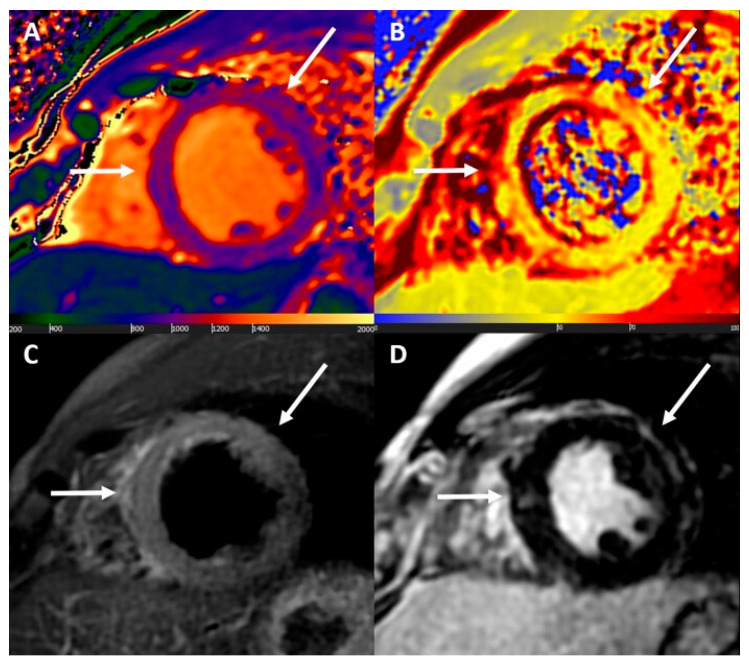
Cardiac magnetic resonance findings. Acute non-ischaemic myocardial injury at mid to apical septal, anterior, and anterolateral walls. (A) Increased native T1 values (up to 1260 ms); (B) increased T2 values (up to 71 ms); (C) increased signal intensity at T2-weighted images; and (D) nonischaemic late gadolinium enhancement. All abnormal findings have matched subepicardial distribution (white arrows). Reproduced with permission from ref. [81].
